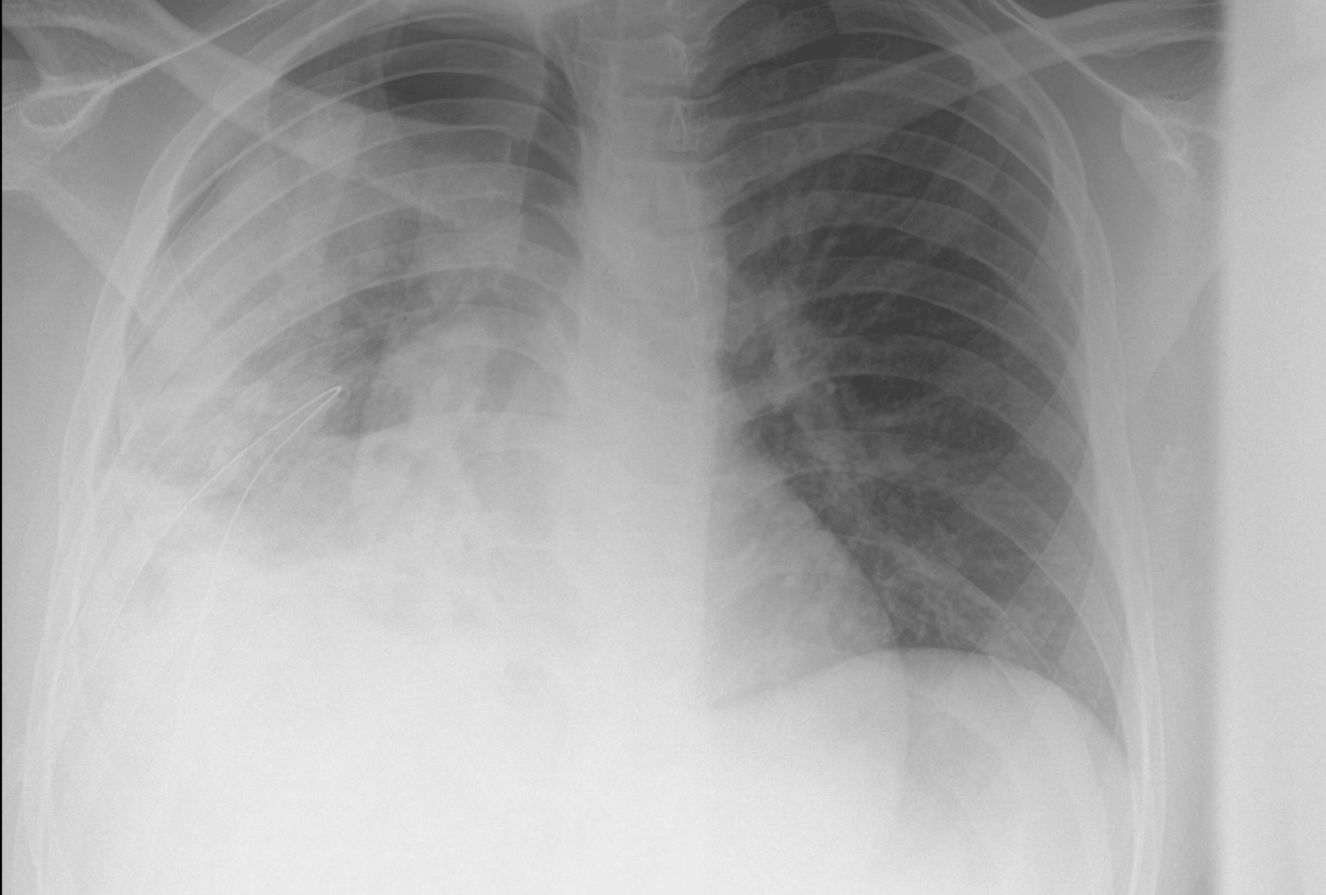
Post-drain chest radiograph showing resolved pneumothorax and residual pleural effusion.
